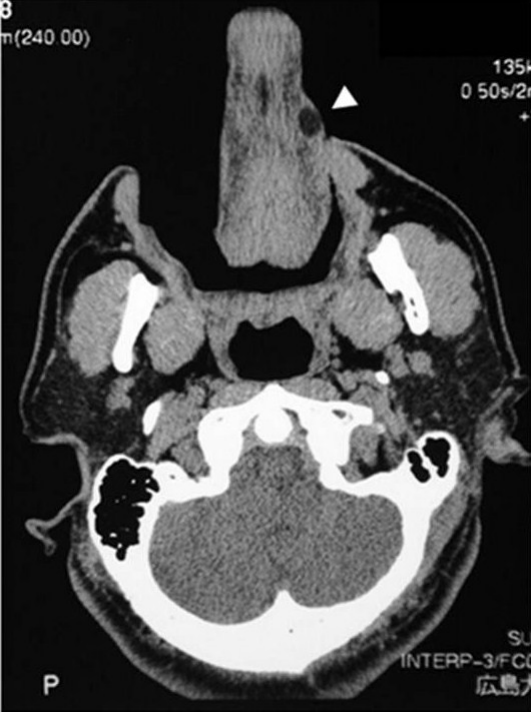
CT revealed a mass at the left side and the edge of the tongue.
